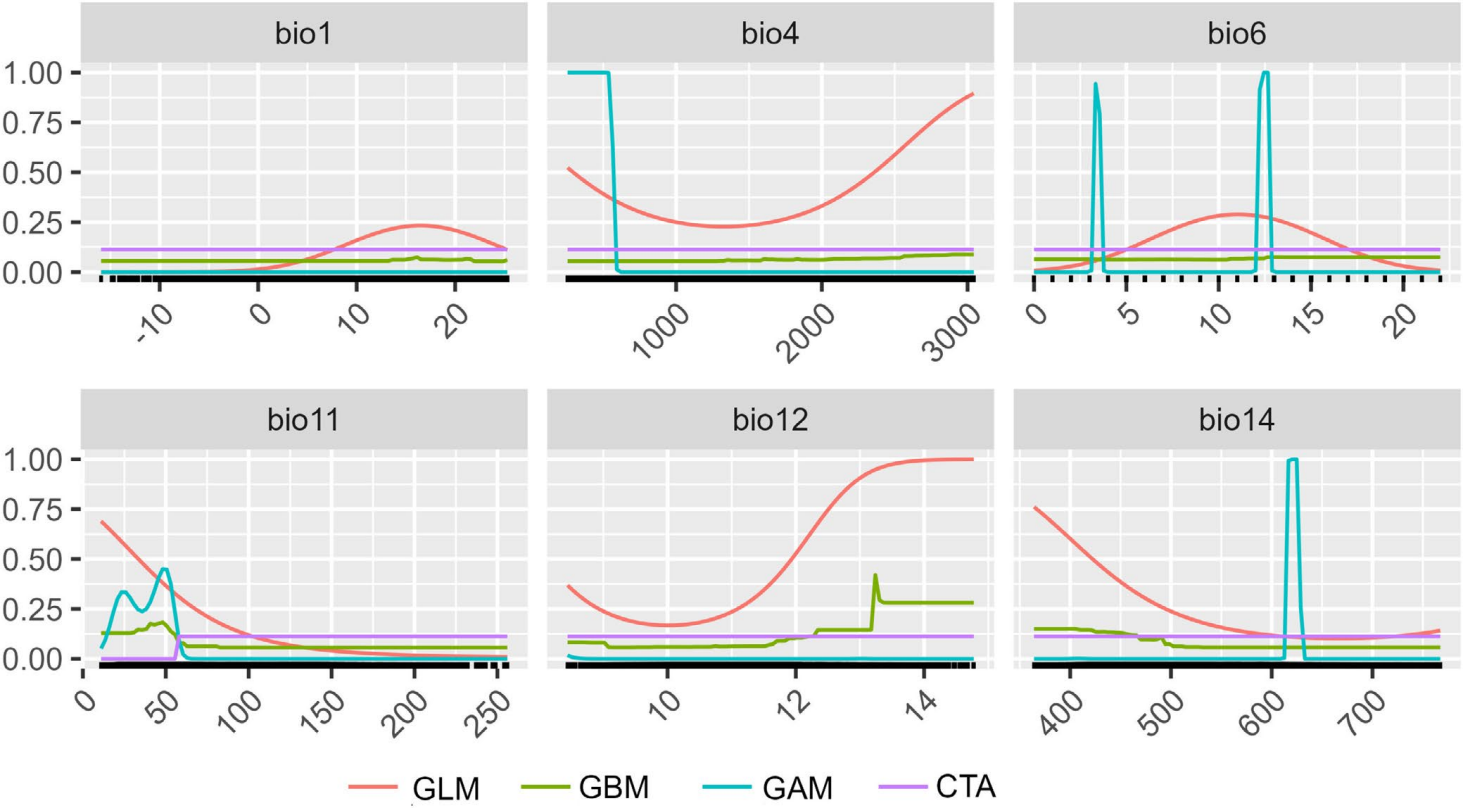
The response curve showing the maximum probability of occurrence of tree‐ferns in different environmental variables: Bio11–mean temperature of coldest quarter, Bio14–precipitation of driest months, Bio1–annual mean temperature, Bio4–temperature seasonality, Bio12–annual precipitation, Bio6–minimum temperature of coldest months in descending order for selected algorithms (GLM, GAM, GBM, CTA).
